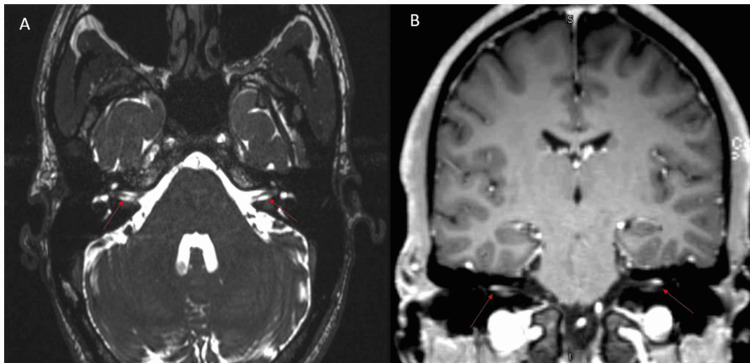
MRI brain with gadolinium. MRI brain axial T2-weighted image of the IAM demonstrating normal caliber facial nerves bilaterally (red arrows in A) and coronal T1 post-contrast image of the IAM demonstrating enhancement of distal intra-canalicular facial nerves (red arrows B). IAM: internal acoustic meatus; MRI: magnetic resonance imaging
